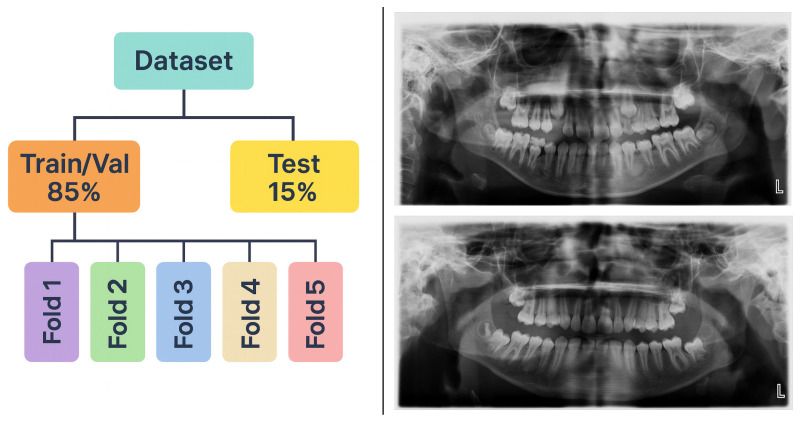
Overview of the dataset split and sample radiographs.
